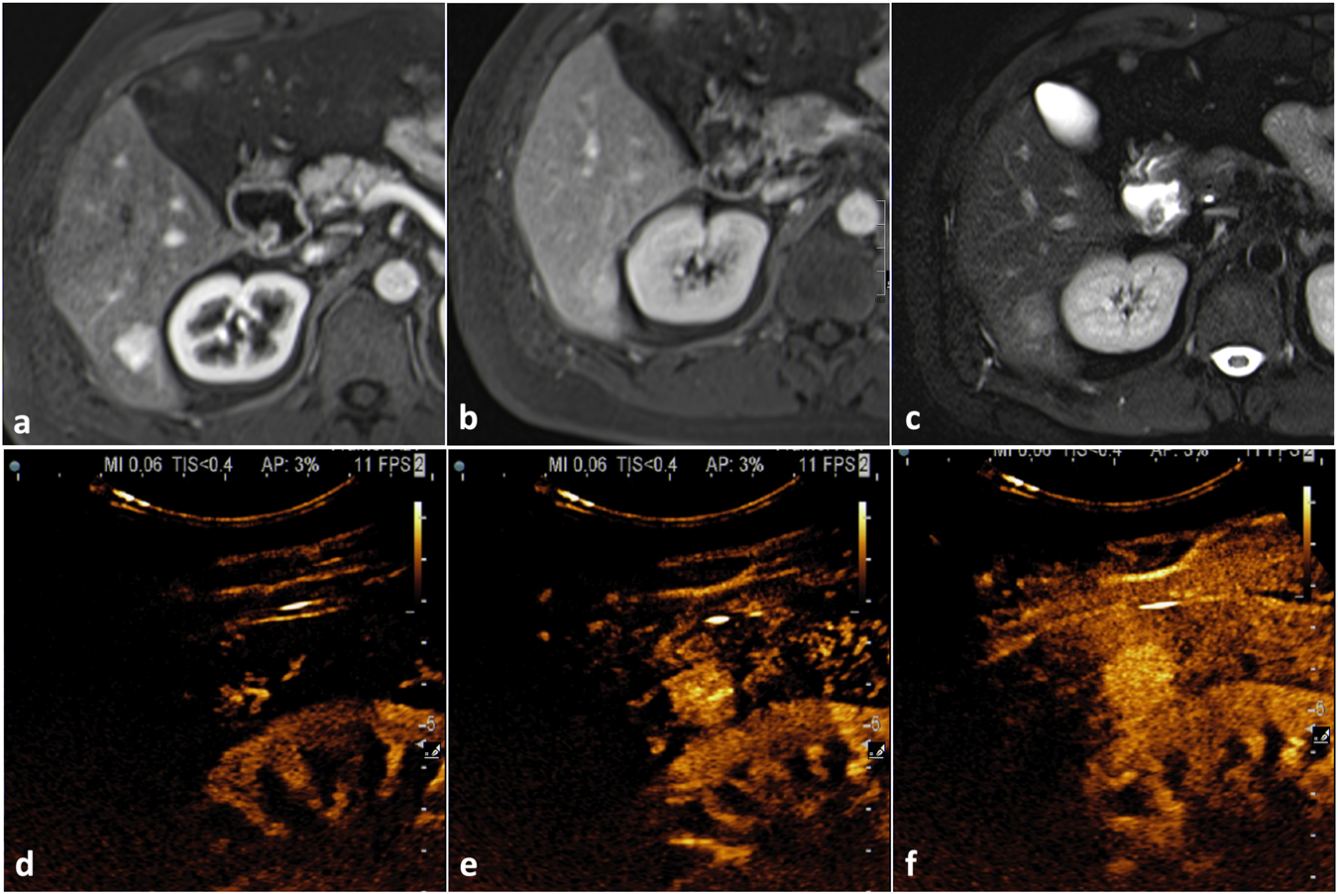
Focal nodular hyperplasia (FNH). The lesion shows strong arterial enhancement (a), but its signal intensity is lower than adjacent vessels in the portal venous phase (b). Moreover, the lesion appears only mildly hyperintense on T2-weighted images (c). On contrast-enhanced US the lesion appears homogeneously hypervascular in the late arterial phase (f ), but rapid centripetal filling can be depicted in earlier arterial phases (d, e)
